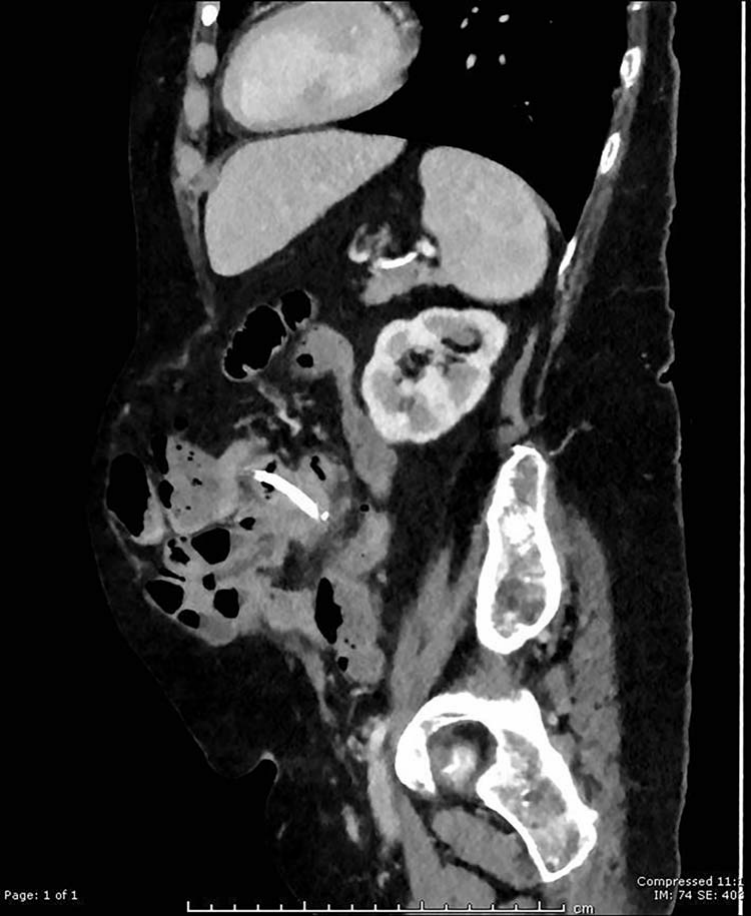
Sagittal CT image showing migrated biliary stent perforation through jejunum located within a large anterior abdominal wall hernia.
